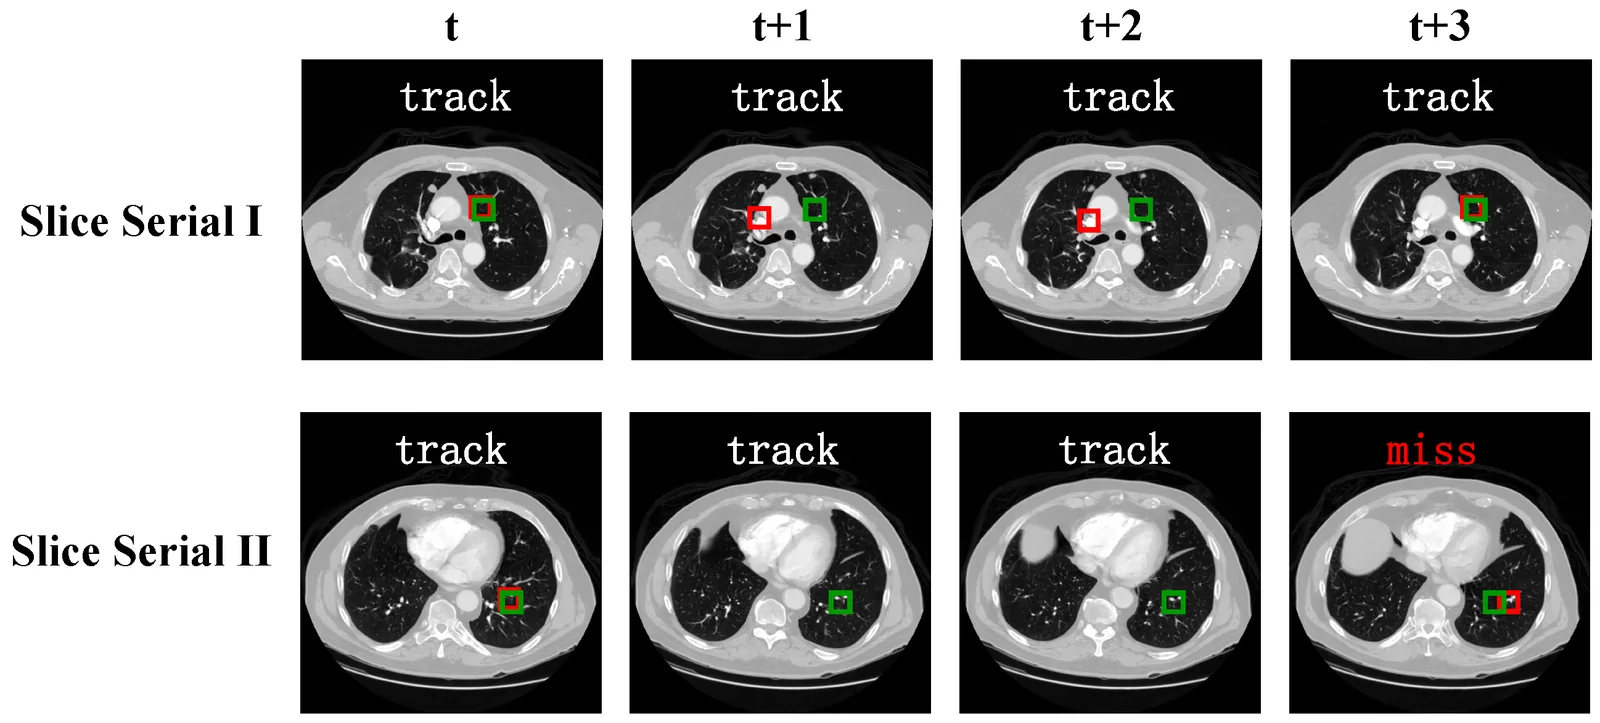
Comparison of centroid distance-based tracking for detected and missed objects. The red boxes denote the raw 2D detection outputs. The green boxes represent the tracked boxes obtained via centroid-distance association. Row I and Row II each show a sequence of four consecutive CT slices. Row I shows a case where the nodule is detected in the first and last slices. The intermediate missed detections (at t+1 and t+2) can be recovered by centroid-distance association. Row II shows a case where the nodule is missed at the sequence boundary (at t+3), making re-identification impossible via distance-based association alone.
